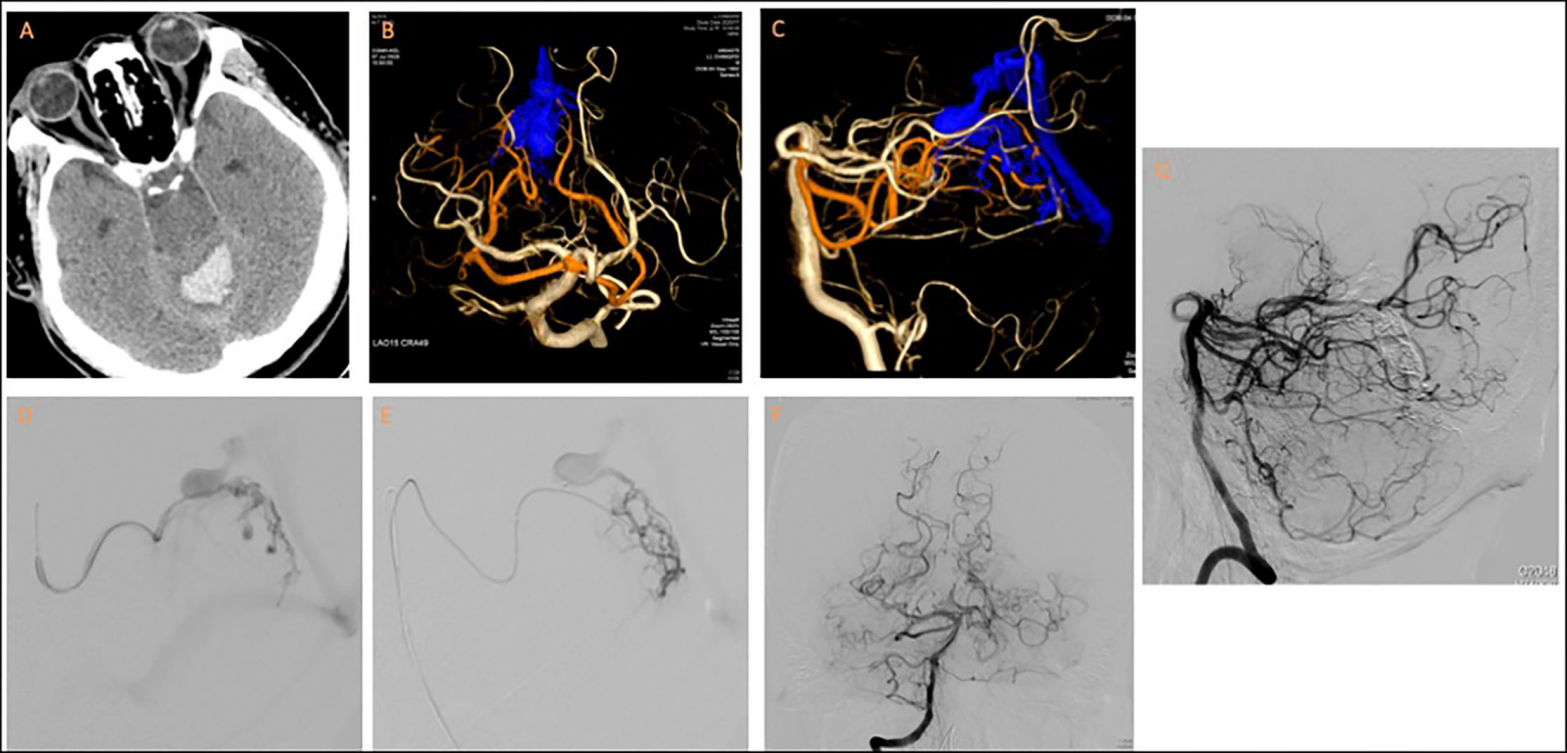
Tentorial dural arteriovenous fistula of case 3. (A) Brain computed tomography revealed fourth-ventricle intraventricular hemorrhage. (B,C) 3D reconstruction of cerebral angiogram revealed the fistula was supplied by medial tentorial branches of bilateral SCAs and drained into vein of Galen through a varix. Notably, a stricture was present at the venosinus junction. Embolization was performed from (D) left and (E) right dural branches of SCAs. Post-embolization angiogram from (F,G) right vertebral artery revealed total obliteration of the fistula.
